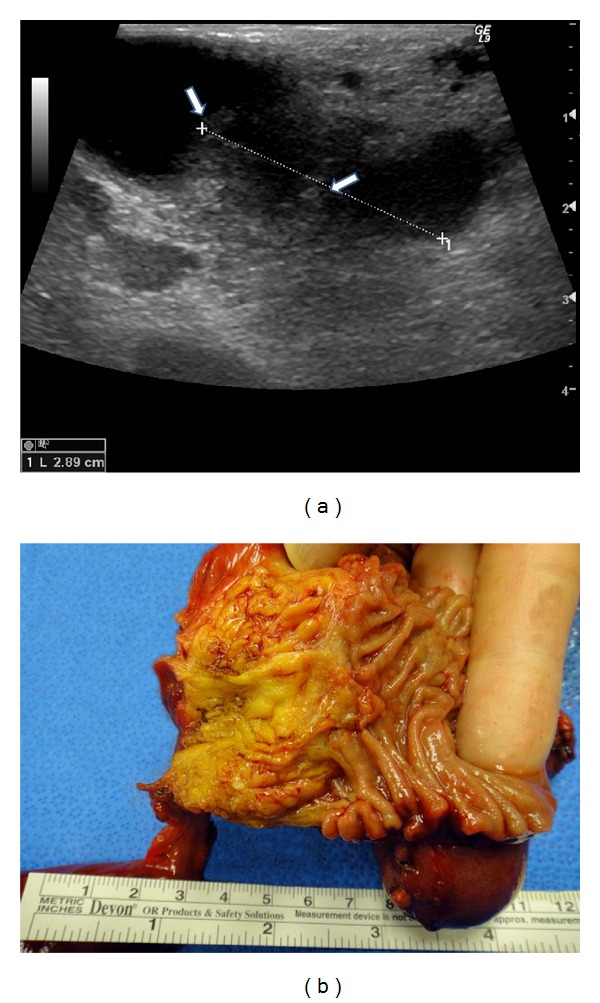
(a) Intraoperative ultrasound demonstrating a dilated common bile duct, diameter 1.3 cm. Note a hypoechoic amorphous mass in the distal lumen of this duct (arrows). (b) Gross picture of the ampullary region showing a 2.0 cm indurated area of the intrapancreatic (distal) common bile duct.
